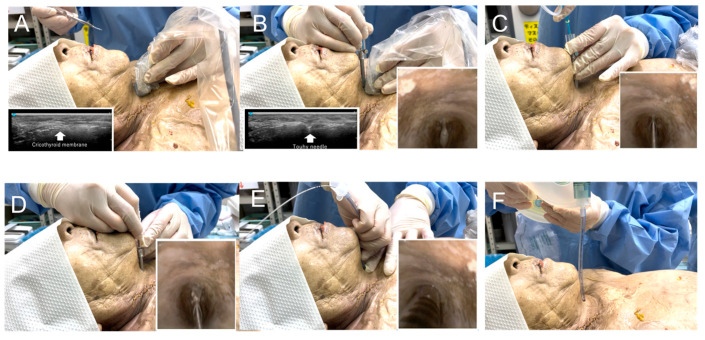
Ultrasound-guided cricothyroidotomy on a cadaver. Images show the cricothyroidotomy performed on a cadaver using an ultrasound-guided cricothyroidotomy kit. Cadavers were fixed in N-vinyl-2-pyrrolidone. Fixation was characterized by the softness and suppleness of the tissues. (A): First, the cricothyroid membrane was identified using ultrasound. (B): The needle was inserted into the trachea through the cricothyroid membrane under ultrasound guidance, and the bronchoscopy image shows the needle in the trachea. (C): Intratracheal needle placement was confirmed by aspirating air using a syringe, after which a guidewire was inserted into the trachea through the needle. (D): The cricothyroid membrane was then dilated using the dilator. (E): After dilation, the needle and dilator were removed, leaving only the guidewire, and the tracheal tube was inserted into the trachea. (F): Ventilation is confirmed by raising the chest wall while squeezing the bag valve mask.
